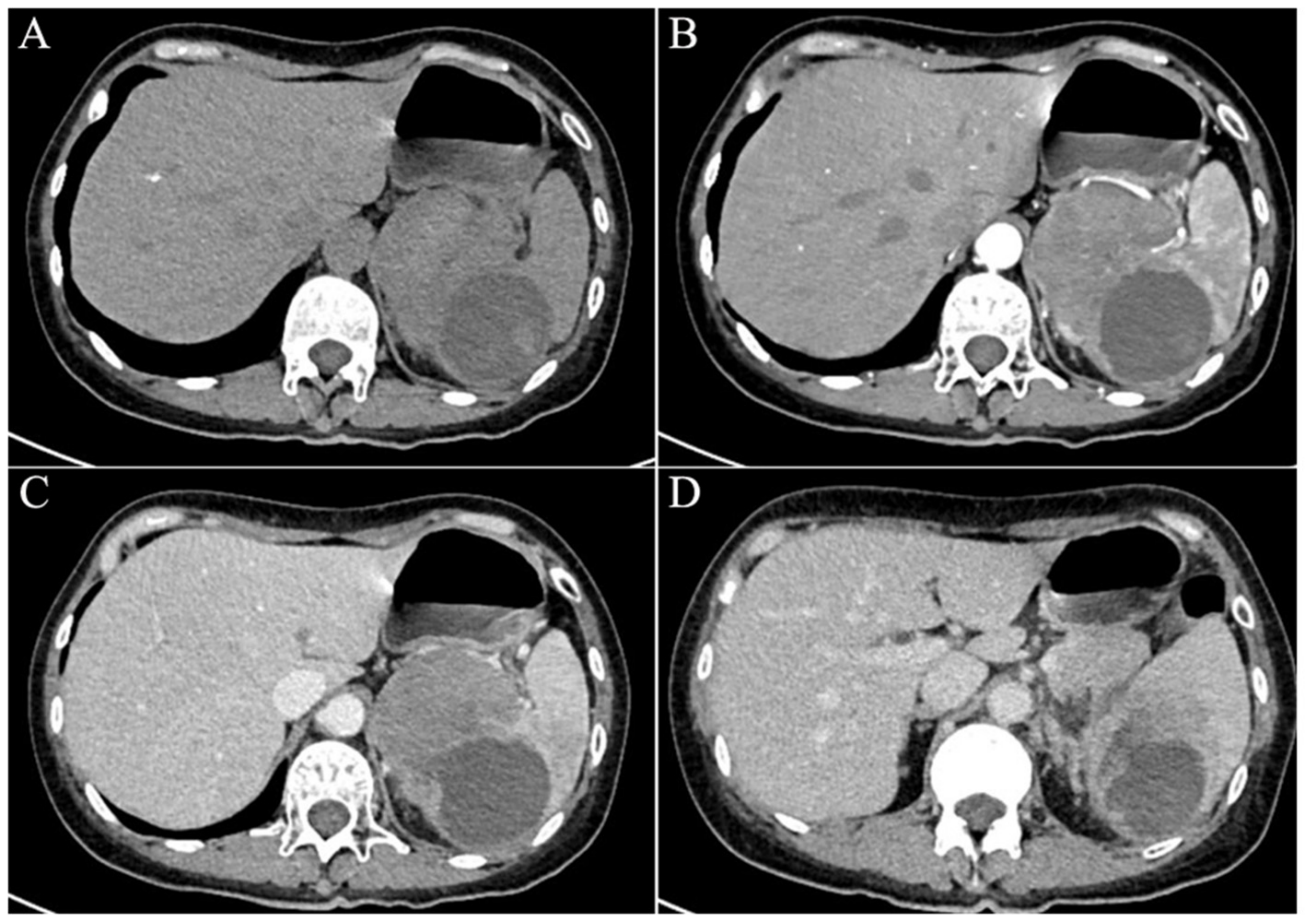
An abdominal computed tomography scan confirmed a 9.5 × 6.5 cm heterogeneous splenic mass with cystic degeneration (A, B), demonstrating extrasplenic extension abutting the gastric fundus and pancreatic tail (C, D).
